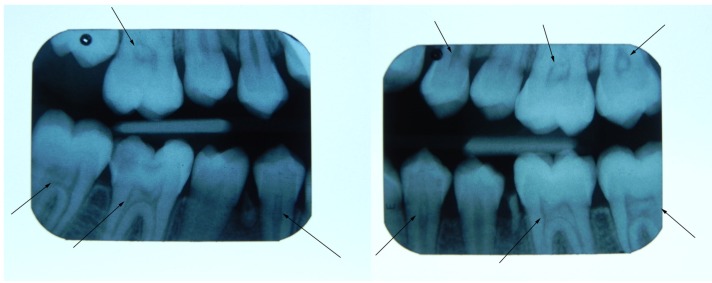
Pulp stone observed inside the pulp chambers of the molars and premolars in the bitewing radiograph.
